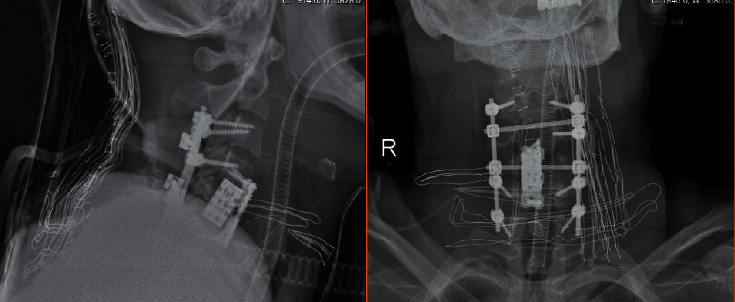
Plain radiograph obtained after surgical repair of cervical injury by a combined anterior and posterior approach.
